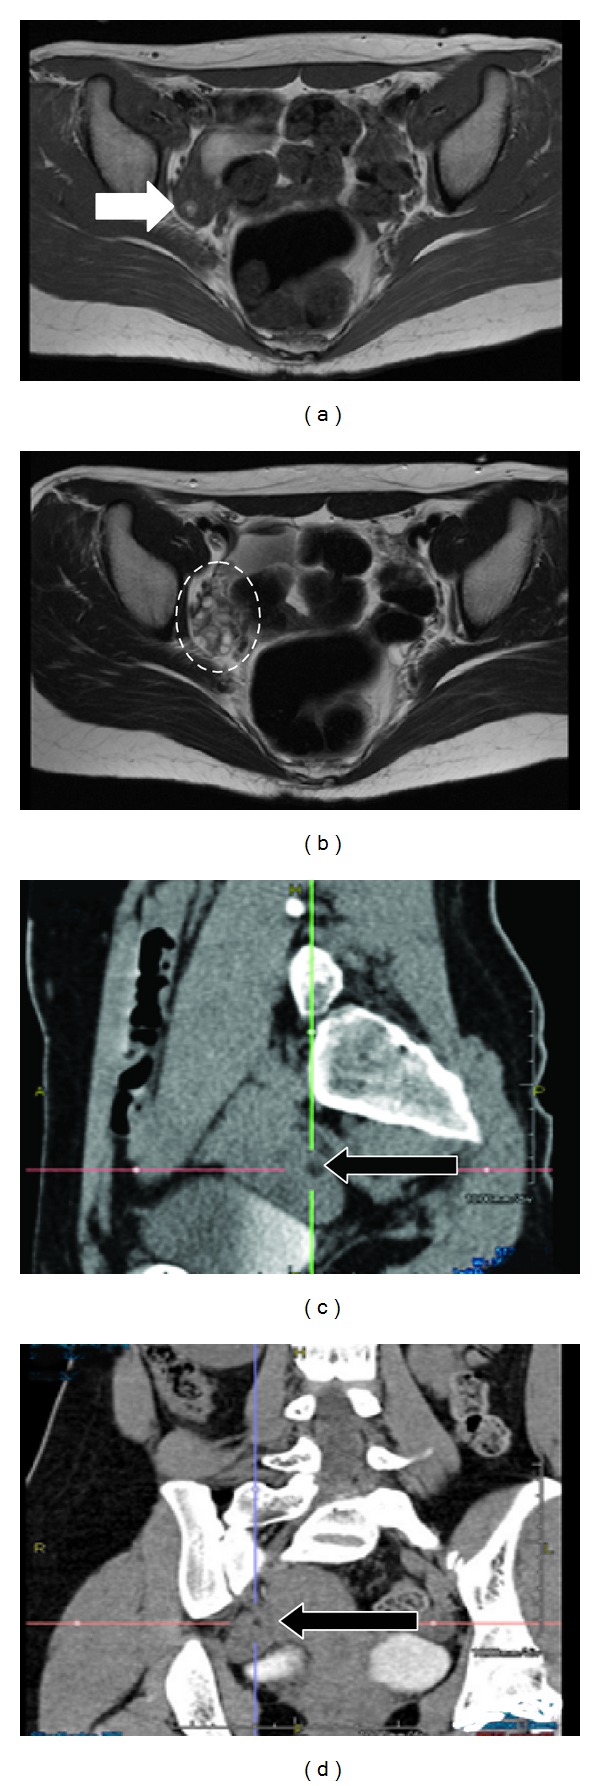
Image diagnosis. (a) T1-weighted transverse pelvic MRI; open arrow indicates a 5 × 7 mm high-intensity area in the right ovary that shows a fat component of the ovarian teratoma. (b) T2-weighted transverse pelvic MRI; broken-lined circle indicates the right ovary, which contains many antral follicles of the same size as the teratoma. ((c), (d)) Abdominal enhanced CT with 1 mm slice thickness in a sagittal plane (c) and in a coronal plane (d); closed arrow indicates a 5 × 7 mm low-density area in the right ovary that shows a fat component of the teratoma. The mean CT number was −62.5 HU.
